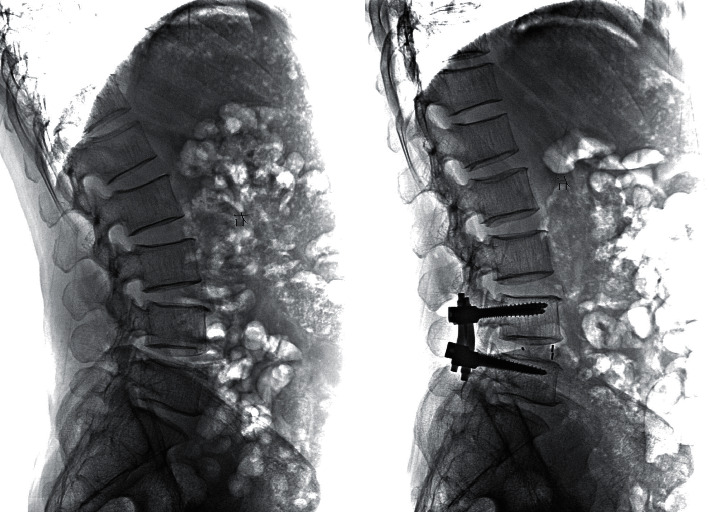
Representative case of a patient with an L4-5 LDH. Preoperative (a) and postoperative (b) radiographs were obtained by ERAS during the operation.
